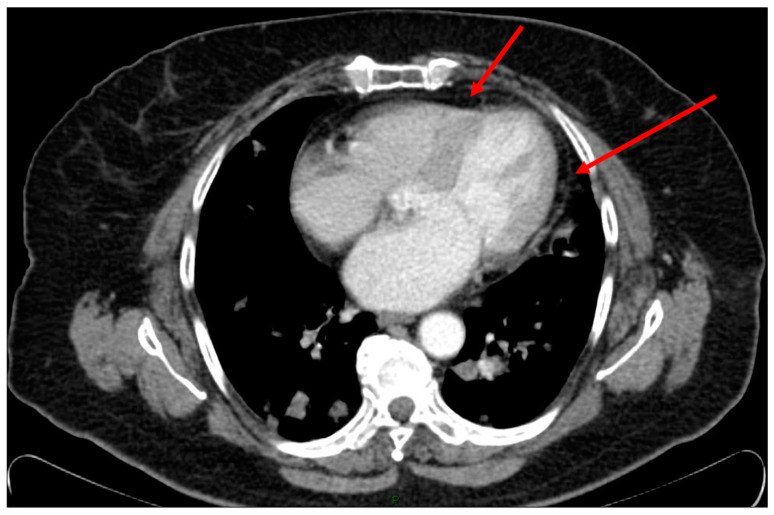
Computed tomography angiography of the chest at presentation, showing the pericardial effusion (the black area near the red arrow).
